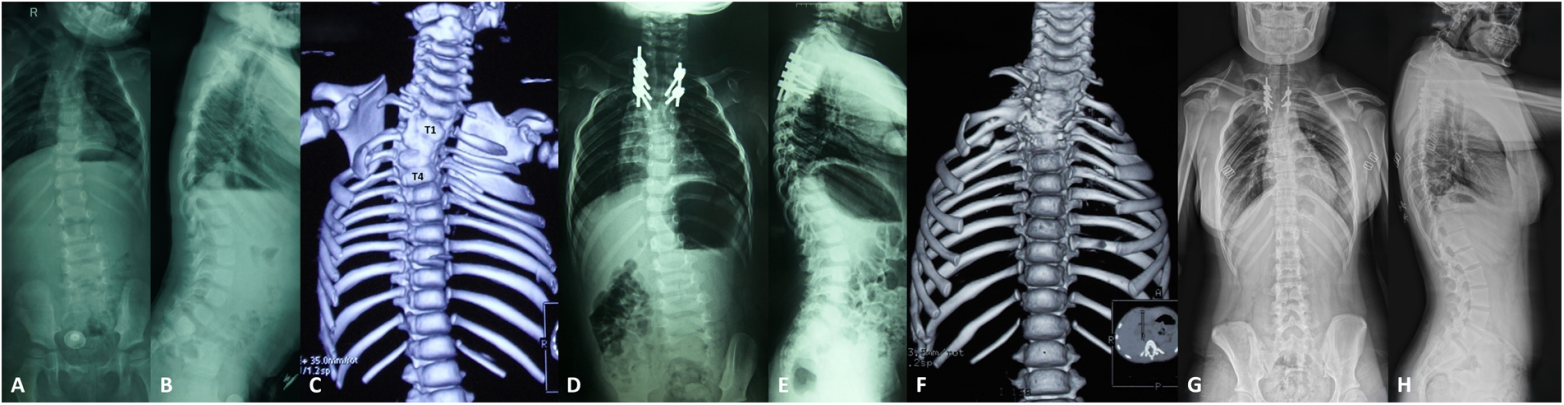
A 2-year-old girl (case 18) with congenital cervicothoracic scoliosis combined with congenital high scapular. (A,B) Preoperative radiographs showed that the segmental scoliosis was 54°, the distal compensatory curve was 25°, the T1 tilt was 30°, the clavicle angle was 14°, and the neck tilt was 24°. (C) Preoperative CT three-dimensional reconstruction showed the left T1-T4 bar. (D,E) Posterior right T2-T3 osteotomy and contralateral bar release combined with pedicle screw internal fixation were performed. Postoperative radiographs showed that the segmental scoliosis was 6° with a postoperative correction rate of 88.9%, the distal compensatory curve was 8°, the T1 tilt was 2°, the clavicle angle was 5°, and the neck tilt was 4°. (F) Postoperative CT demonstrated a solid fusion. (G,H) At the 15-year follow-up (the patient was 17 years old), the correction was maintained well, and no significant correction loss was observed during the follow-up.
